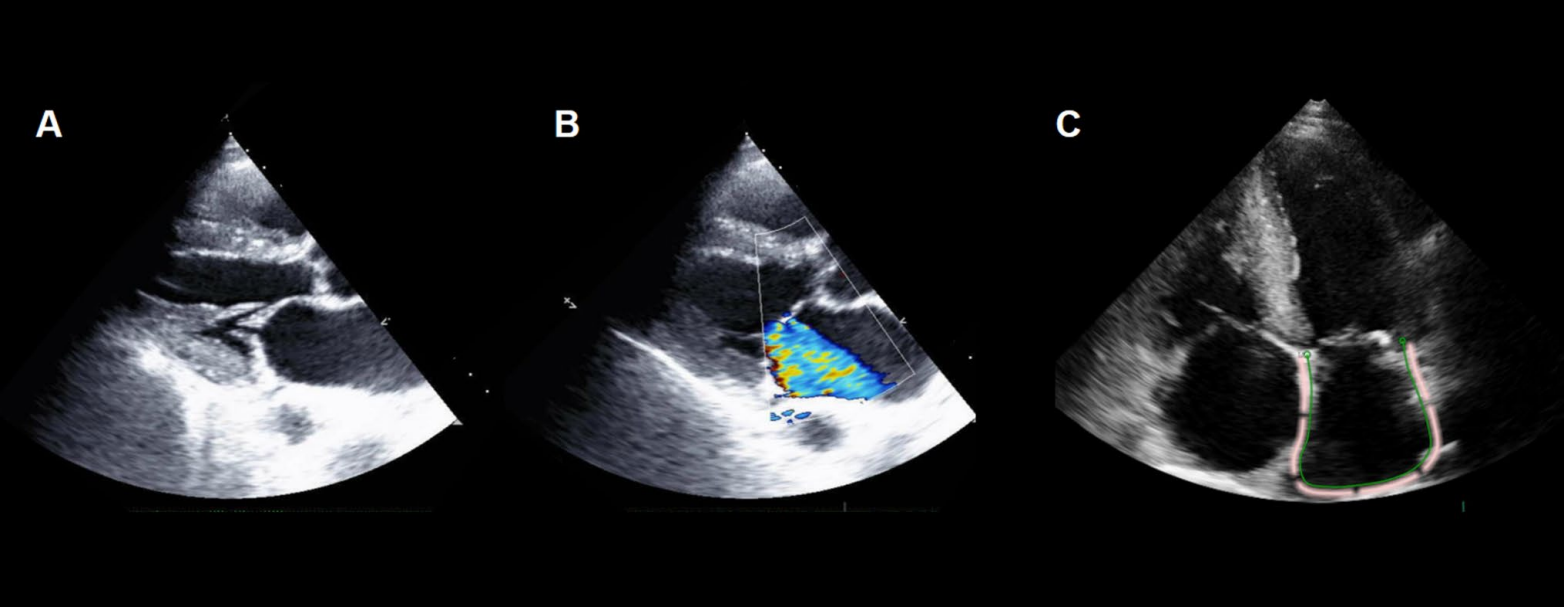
Echocardiographic features of mitral regurgitation (MR). Features of MR in a 50-year-old man with amyloid light-chain cardiac amyloidosis: elongated and thickened leaflets and subvalvular apparatus (A), massive regurgitation (B), left atrial turbulence with reduced function (peak atrial longitudinal strain 5%)
